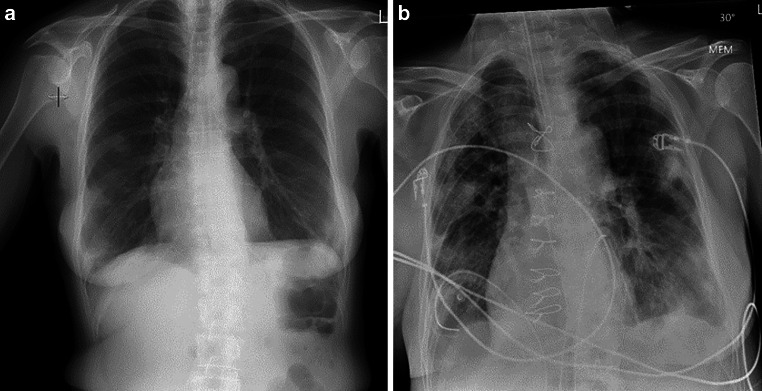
a Routine X‑ray before brachytherapy showing patchy alterations in both lungs; b X-ray one day after cardiac surgery
